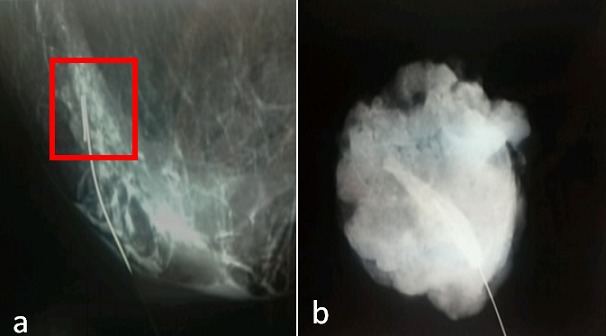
(a): radiographie de la lésion repérée par Harpon; (b): radiographie de la pièce après tumorectomie
